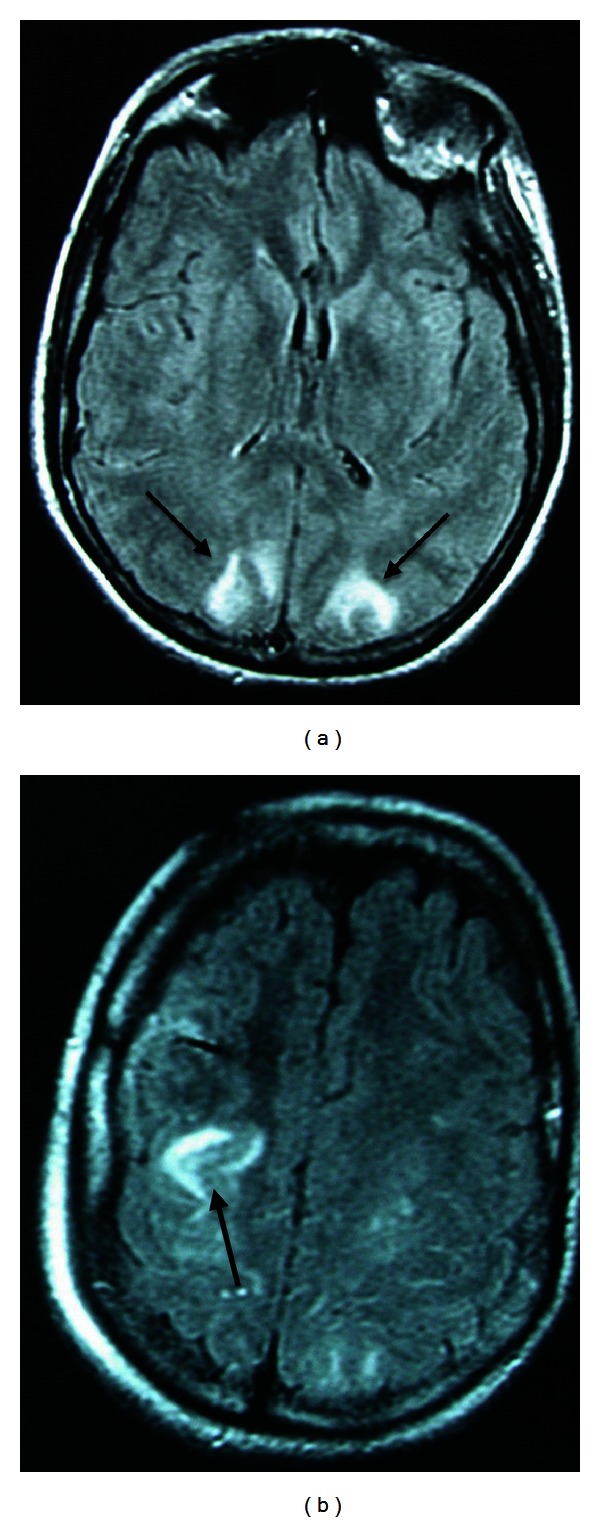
(a) Fluid attenuated inversion recovery magnetic resonance imaging indicating high signal lesions on both occipitals lobes. (b) Fluid attenuated inversion recovery magnetic resonance imaging indicating high signal lesions on the right parietal lobe.
